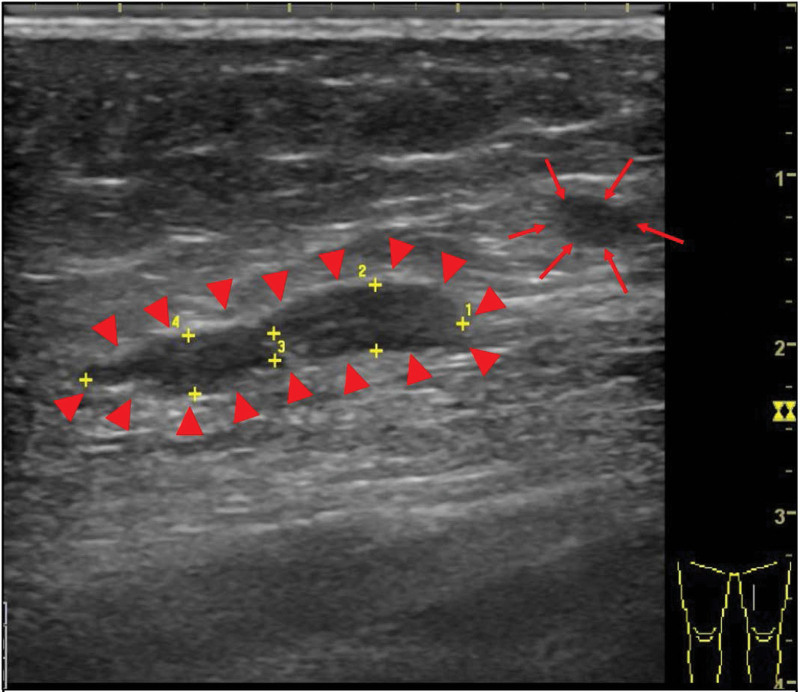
The preoperative sonography before first autograftectomy showed 2 hypoechoic masses. The larger one (1.7 cm × 0.4 cm, arrow head) and the smaller one (0.6 × 0.4 cm, arrow) at adjacent area.
